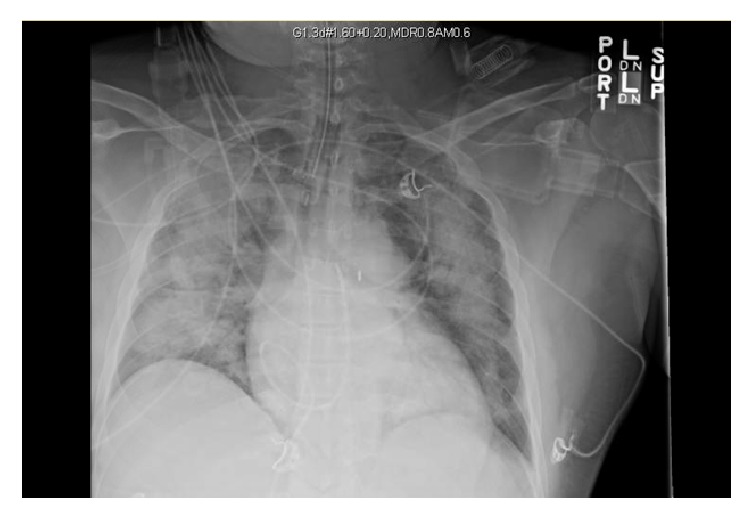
Portable chest X-ray, supine.
